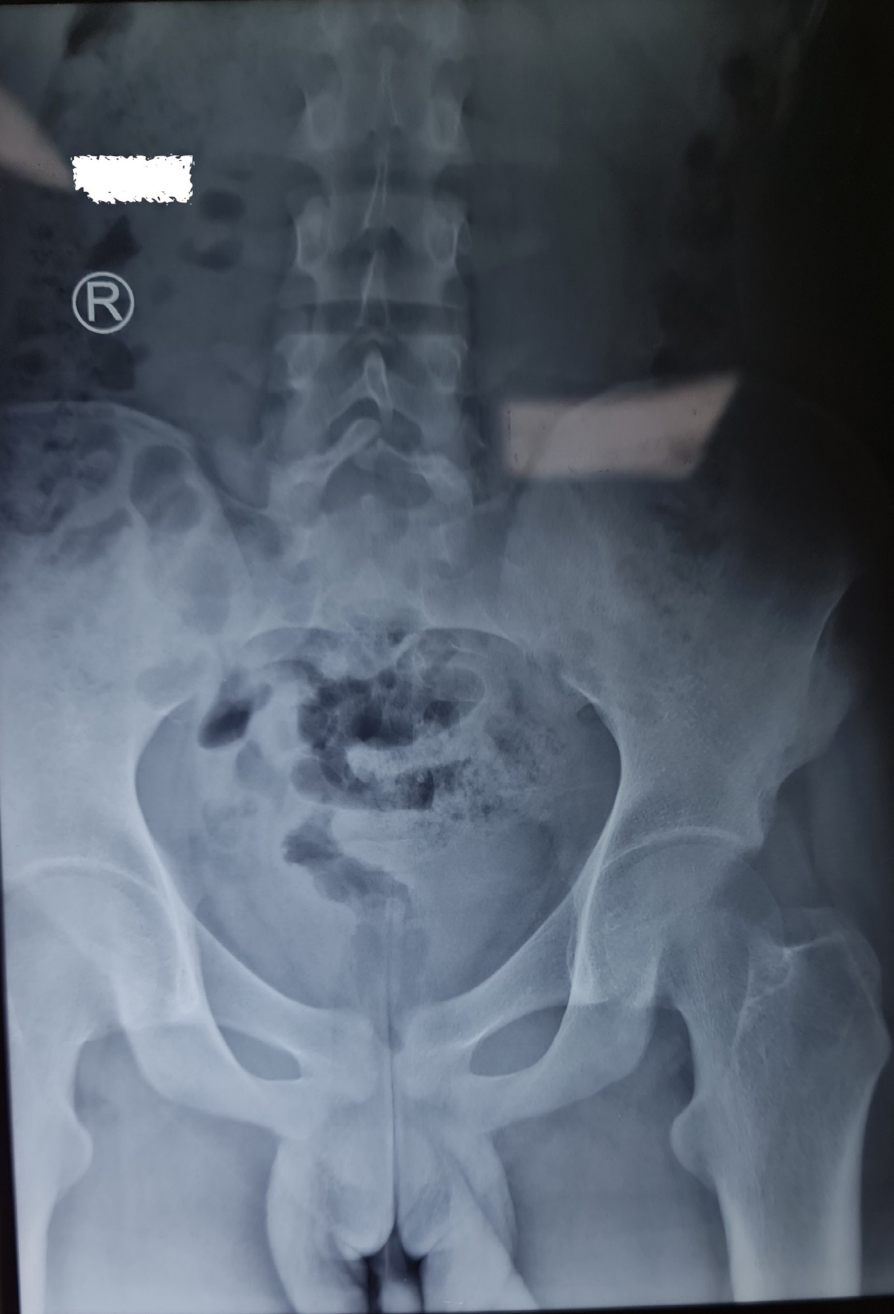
A pelvic radiograph revealed grade 3 sacroiliitis.
